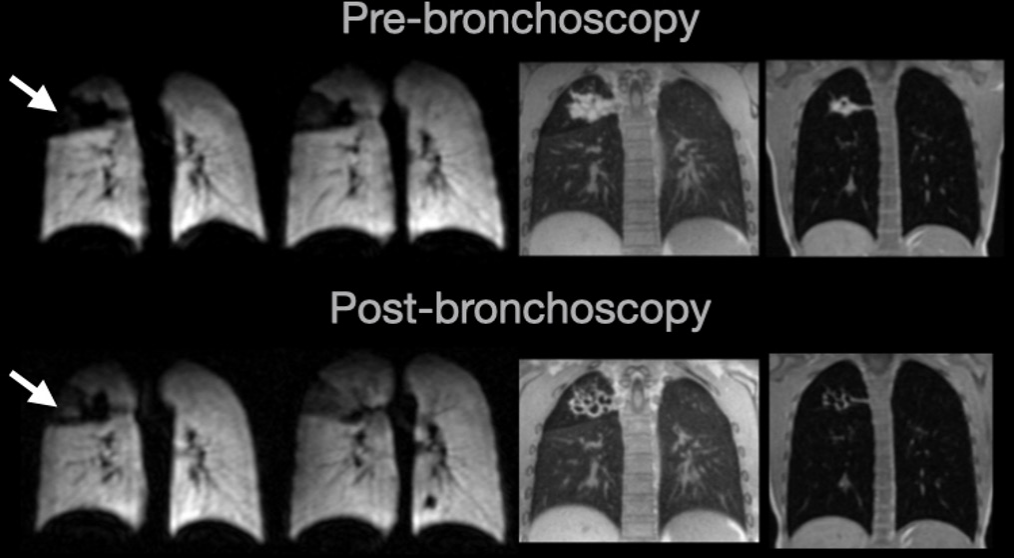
Patient E: clinical diagnosis of CF monitored longitudinally. At the patient’s routine clinical review, a chest X-ray and CT showed a right-upper lobe collapse and the patient was diagnosed with ABPA. After standard clinical management, a repeat CT scan at 6 months was performed, which still showed the right-upper lobe collapse. At this stage, the patient had never reported any clinical symptoms and spirometry had always remained unchanged at 90—95% predicted. The patient was therefore referred for MRI assessment of both ventilation and structure. A large ventilation defect was present in the right-upper lobe on ventilation MRI and did not ventilate at TLC, suggesting complete obstruction. ^1^H MRI images depicted the cause of the ventilation defect; a large mass of mucus-filled airways in the right-upper lobe. Upon reviewing these images, the clinical team decided to perform a bronchoscopy and repeat the MRI assessment. Repeat MRI was performed approximately 2 weeks post-bronchoscopy and showed that the large ventilation defect remained at end inspiratory tidal volume, but with some improved ventilation compared with pre-bronchoscopy, especially at TLC. ^1^H structural MRI demonstrated the removal of a large mass of mucus, though the mucus-affected airways remained damaged and functionally impaired. This case study highlights the complementary nature of ^129^Xe functional and ^1^H structural MRI and their promise in clinical assessment of bronchoscopy success as an alternative to repeat CT, especially when spirometry has limited clinical value. Key: from left-to-right; ^129^Xe ventilation MRI at end inspiratory tidal volume, ^129^Xe MRI at TLC, ^1^H ultra-short echo MRI, ^1^H spoiled gradient echo MRI at TLC. ABPA, allergic bronchopulmonary aspergillosis; CF, cystic fibrosis; TLC, total lung capacity.
